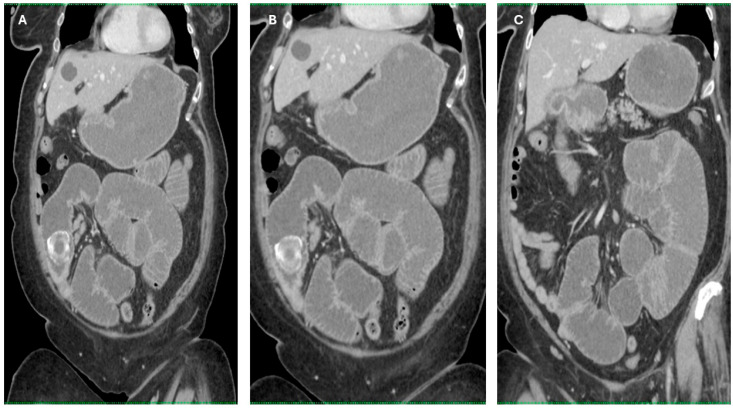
Enteric gallstone associated with cholecystoduodenal fistula. Coronal reconstructed CT images showing an ectopic gallstone within the intestine, secondary to a cholecystoduodenal fistula—a classic radiological finding in biliary ileus. (A–C) Coronal reconstructions showing multiple distended small bowel loops upstream from the obstruction site, with the intraluminal gallstone visible in the terminal ileum. Acquisition with 5 mm slice thickness and 3 mm reconstruction. Images have been cropped and zoomed for optimal visualization while maintaining the original scale; a 10 mm scale bar is included in each panel.
